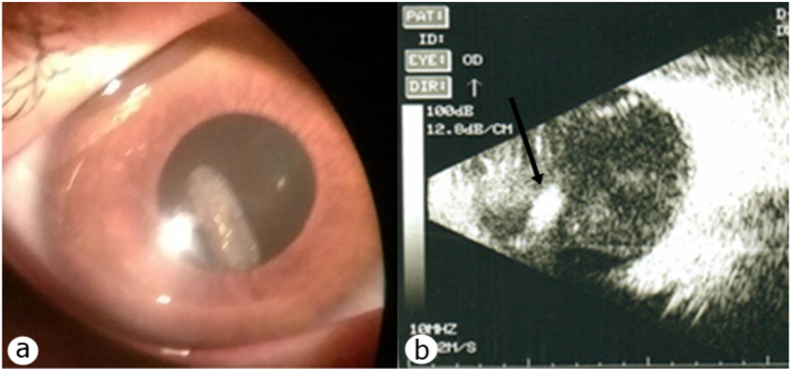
Case №2. a. On slit-lamp examination, a larva is visualized in the vitreous posteriorly to the lens capsule. b. Ocular ultrasound demonstrating a larva (black arrow).
